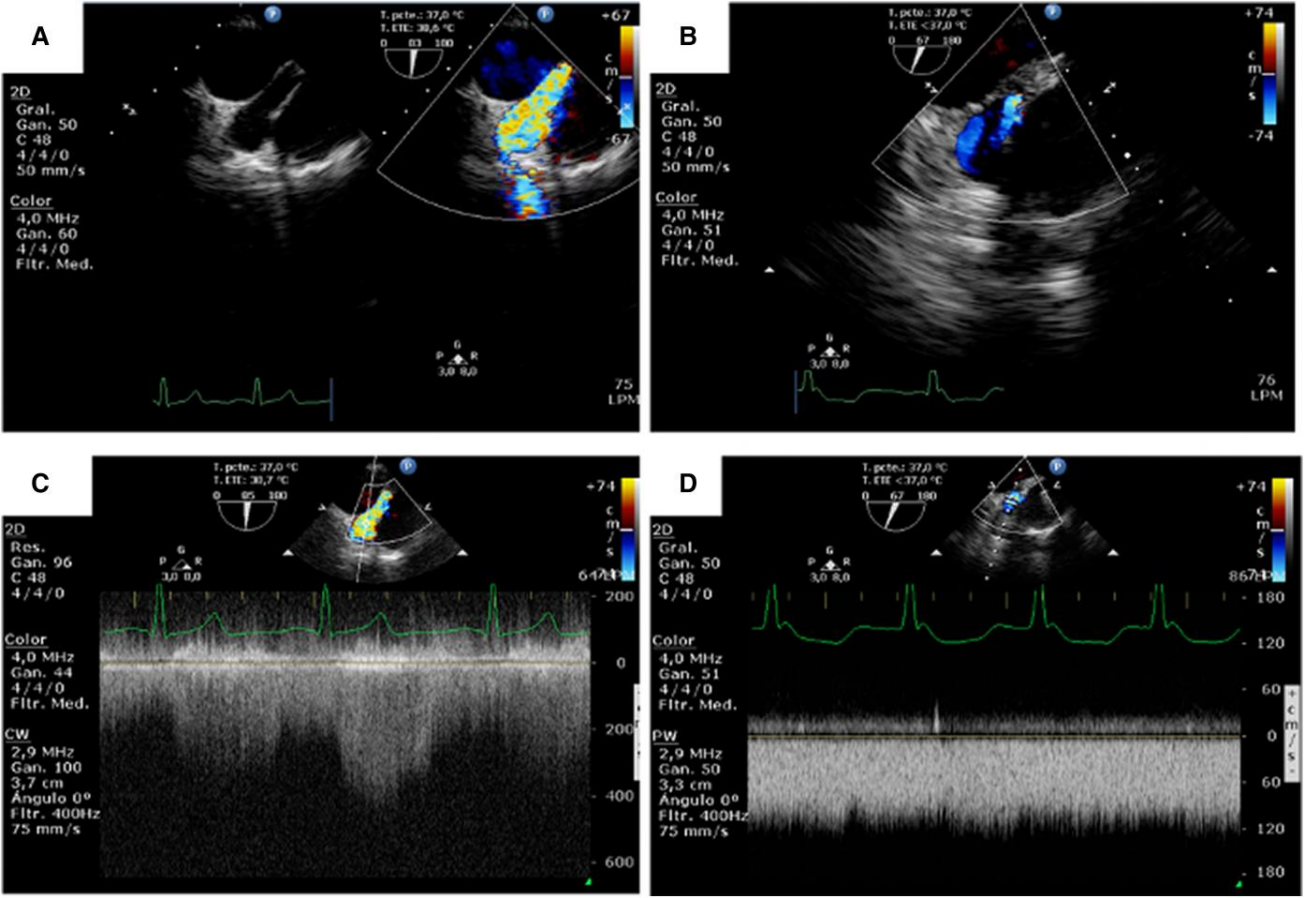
Echo Doppler of the fistulous tract pre- (*A*, *C*) and post-surgery (*B*, *D*). (*A*) Turbulent flow in the fistulous tract. (*C*) Pulsatile flow inside the fistulous tract. (*B*) Absence of the turbulent flow in the fistulous tract. (*D*) Laminar flow inside the fistulous tract.
